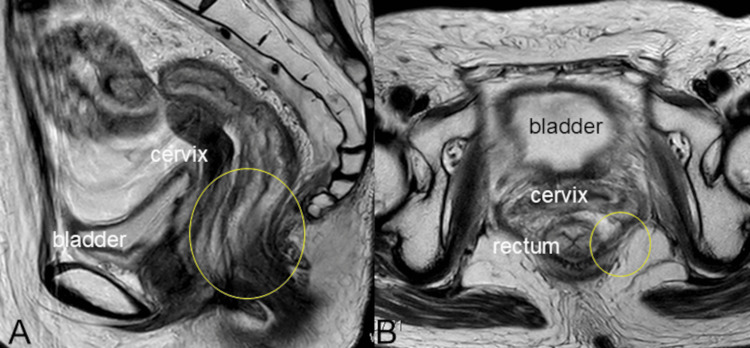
MRI images after weekly paclitaxel A Sagittal section of the MRI images after weekly paclitaxel; B Horizontal section of MRI images after weekly paclitaxel
